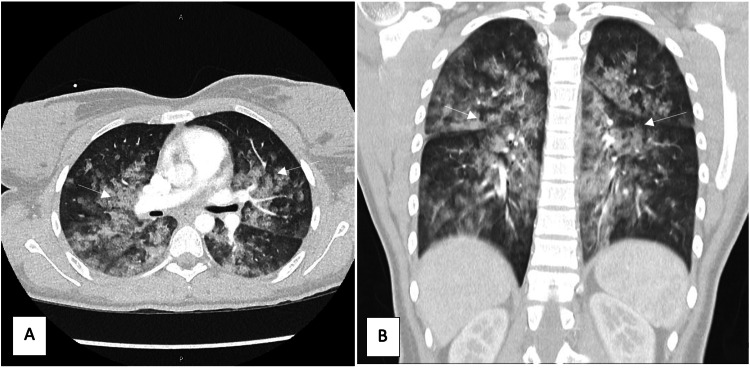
A: Axial cross-section of the chest CT; B: Coronal cross-section of the chest CT Images obtained following admission to the hospital for further observation. Patchy ground-glass opacities are present bilaterally with confluence at the central and hilar regions. Findings are worrisome for alveolar edema.
